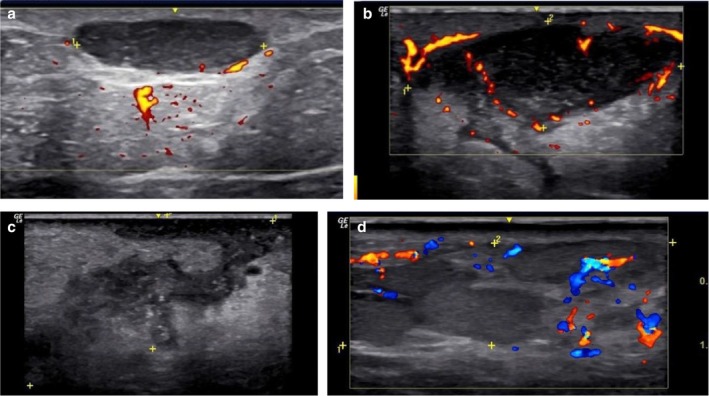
(a) Stage I (granulomatous phase): Hypoechoic nodular area with increased echogenicity of the subcutaneous tissue (panniculitis) and moderate intralesional and peripheral vascularity on Doppler. (b) Stage II (liquefaction phase): Hypoechoic heterogeneous collection with internal echoes, increased echogenicity of the subcutaneous tissue (panniculitis), and central and peripheral vascularity. (c) Stage III (tunnelization phase): Interconnected hypoechoic sinus tracts with heterogeneous internal echoes, increased echogenicity of the subcutaneous tissue (panniculitis), and predominantly peripheral vascularity. (d) Stage IV (mixed phase): Interconnected hypoechoic sinus tracts with heterogeneous internal echoes, increased echogenicity of the subcutaneous tissue (panniculitis), and a hypoechoic nodular.
